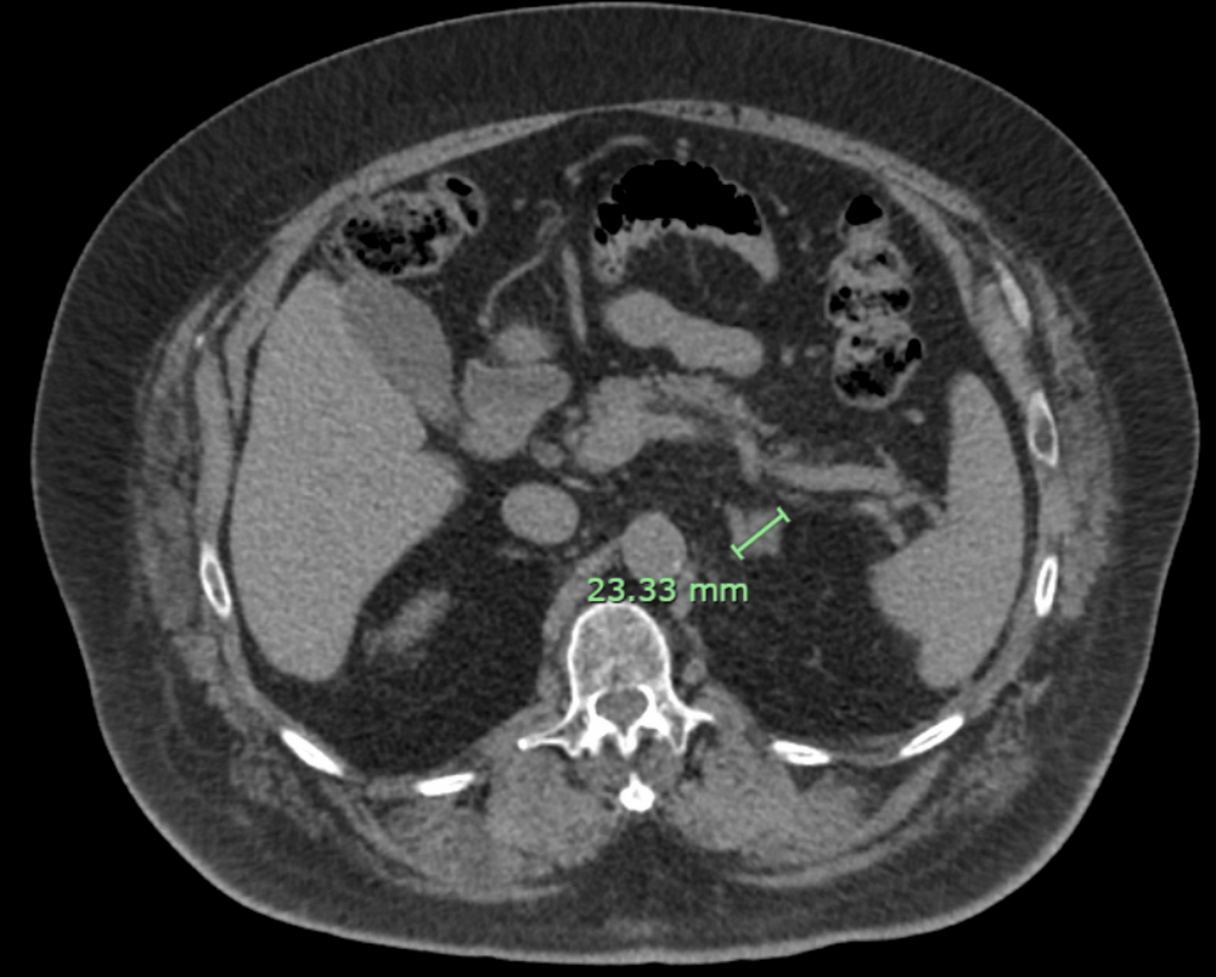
One-year follow-up abdominal CT 3-Phase showing an interval decrease in tumor size to 2.3 cm
